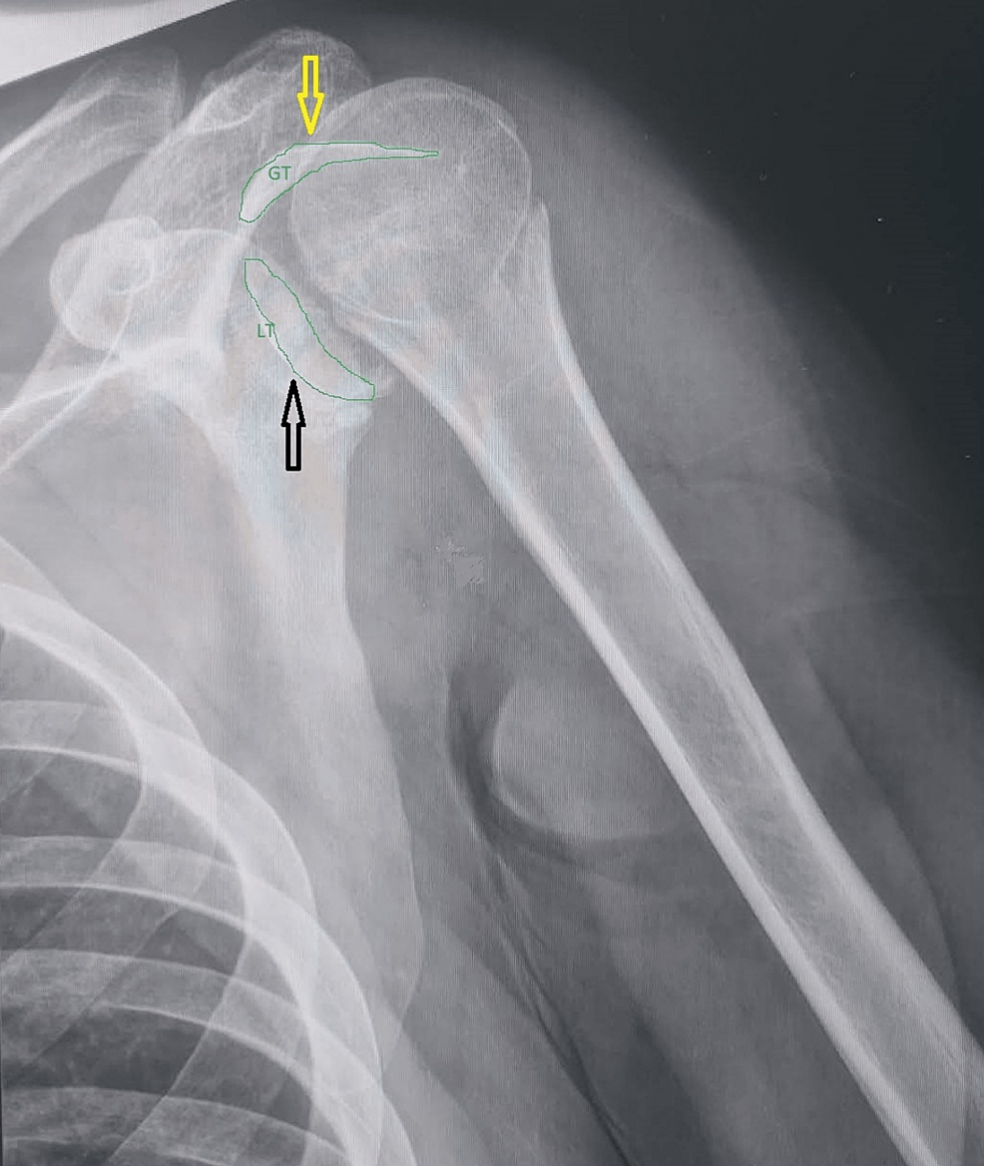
Anteroposterior shoulder X-ray. The greater tuberosity and lesser tuberosity are fractured and displaced. The humeral head is on the same axis as the metaphysis because of the internal rotation (light bulb sign), indicating a possible posterior dislocation. Black arrow: lesser tuberosity. Yellow arrow: greater tuberosity.
